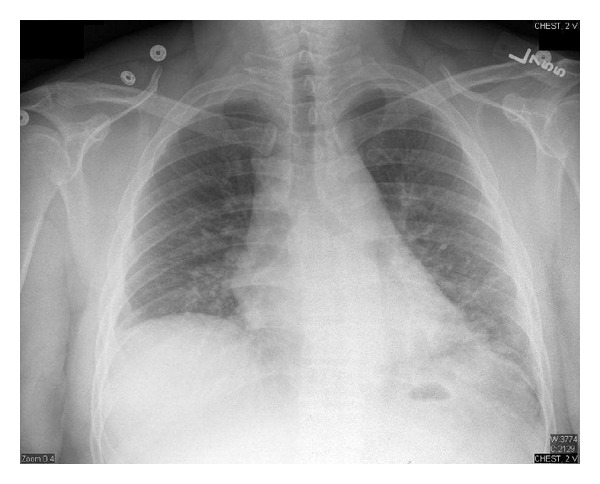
Chest X-ray on day 14 after chemotherapy (ANC was less than 100 cell/*μ*L).
